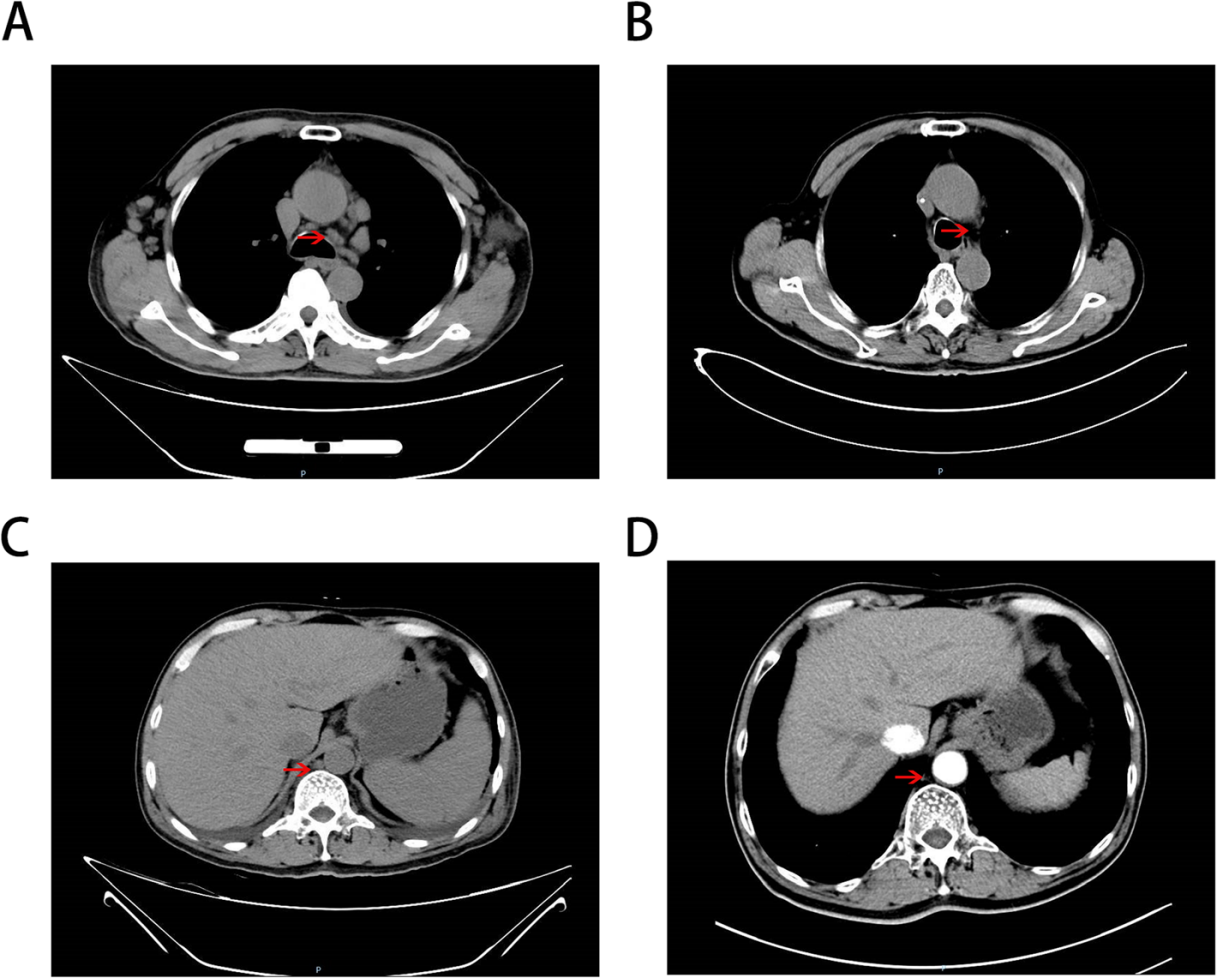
The chest and abdominal computed tomography (CT) a Revealed bilateral axillary and mediastinal lymphadenopathy (arrow), one of which was about 13 × 18mm in the mediastinum before treatment. b Showed lymph node was significantly shrink after treatment (arrow). c Revealed para-aortic and para-splenic lymphadenopathy (arrow), one of which was about 16mm before treatment. d Revealed no obvious lymphadenopathy after treatment (arrow)
